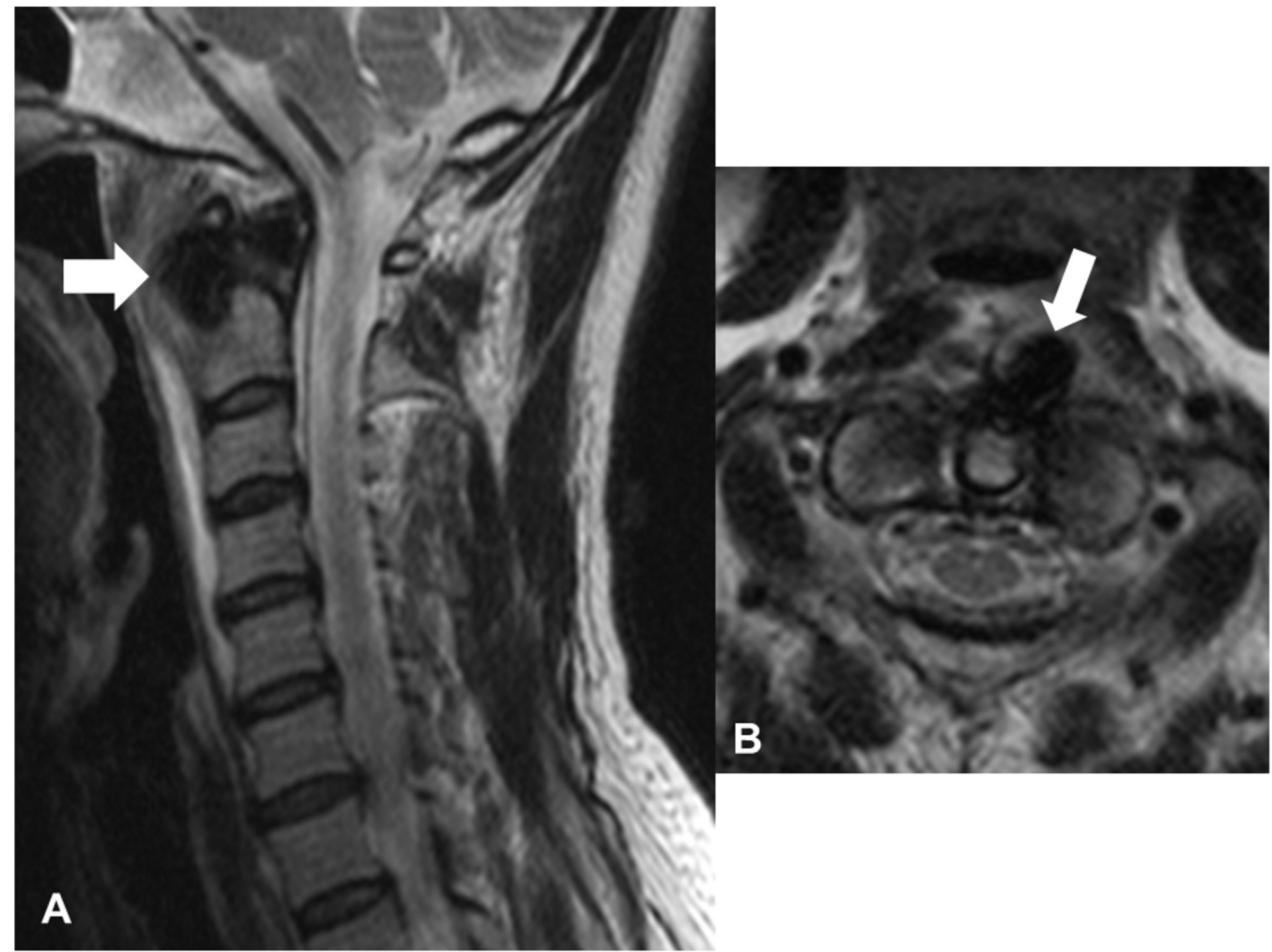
Magnetic resonance imaging of the cervical spine on initial presentation (A) Sagittal and (B) Axial T2-weighted images showing increased signal intensity in the retropharyngeal space between C1 and C5 (arrows)
